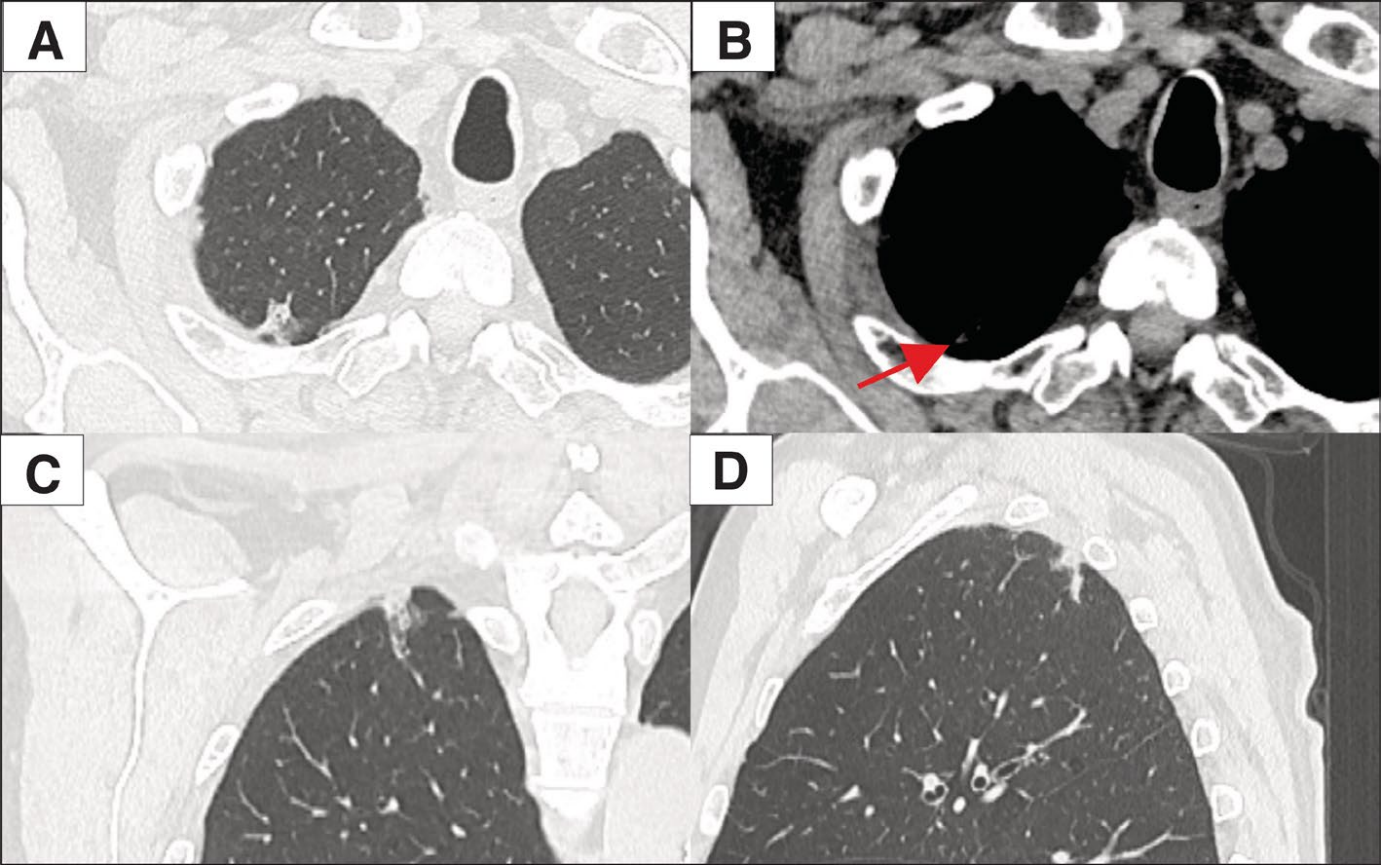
CT findings of a case of alveolar collapse in the preinvasive lesion, which contributed to the preoperative misdiagnosis of clinical stage IA1 lung cancer. Alveolar collapse is observed as a consolidation area in the mediastinal window (arrow). (A) View of the axial lung window, (B) axial mediastinal window. (C) Coronal view, (D) sagittal view.
